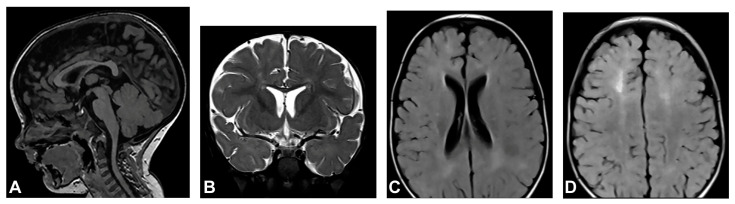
(Case 1) (A) Sagittal T1 MPRAGE magnetic resonance image at the age of two shows macrocrania, thinning of CC and a pineal gland cyst. (B) Coronal T2W magnetic resonance plane shows mild atrophy of bilateral frontal white matter with dilated extracerebral liquor spaces. (C,D) Axial T2 FLAIR magnetic resonance planes show that lateral ventricles are slightly dilated with gliotic changes in periventricular and frontal regions.
